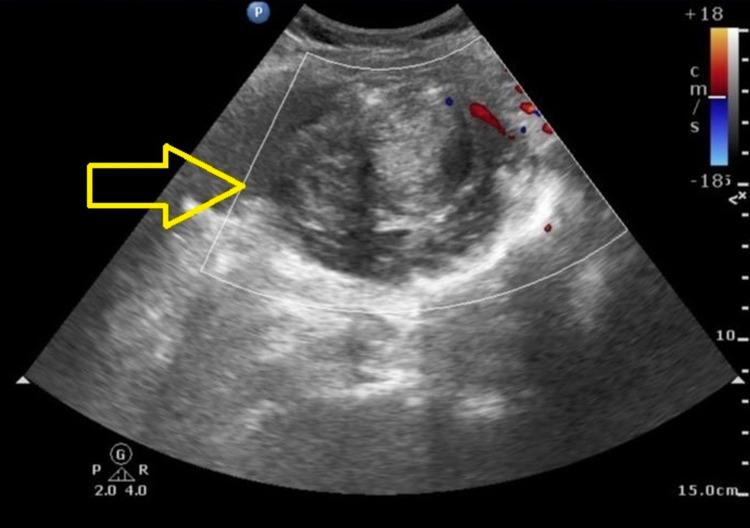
Duplex ultrasound image showing sclerosed pseudoaneurysm of left IEA a minute after thrombin injection.
